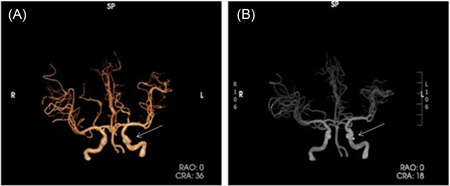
Computed tomography (CT) angiography imaging and revascularization. The patient's CT angiography imaging (A, arrow) and revascularization (B, arrow) of the carotid arteries showed a few calcified spots in the wall of the siphon segment of the left internal carotid artery, and no significant lumen stenosis was observed. [Color figure can be viewed at wileyonlinelibrary.com]
